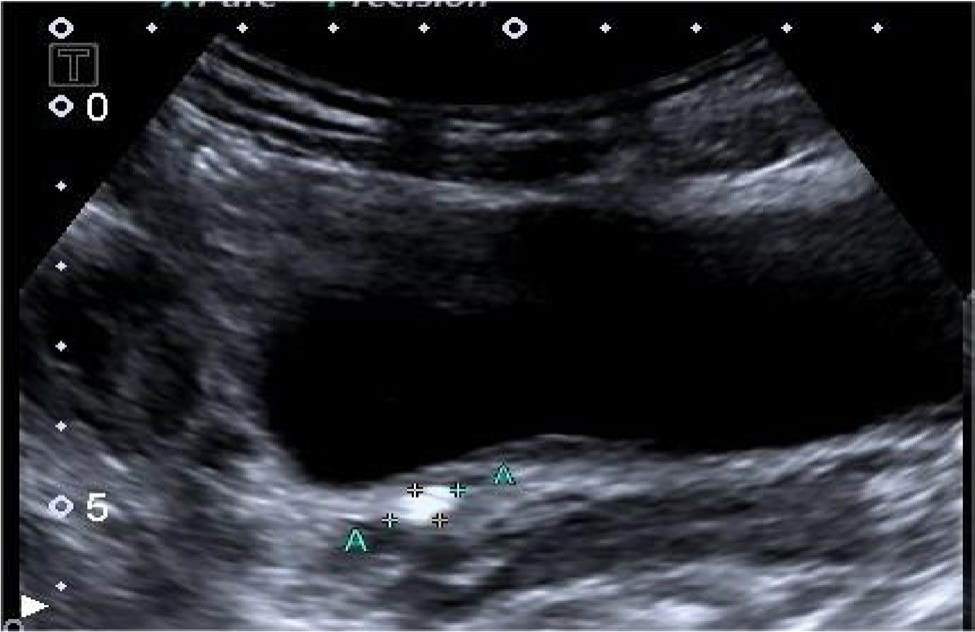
Ultrasound image of ureterovesical junction calculi
